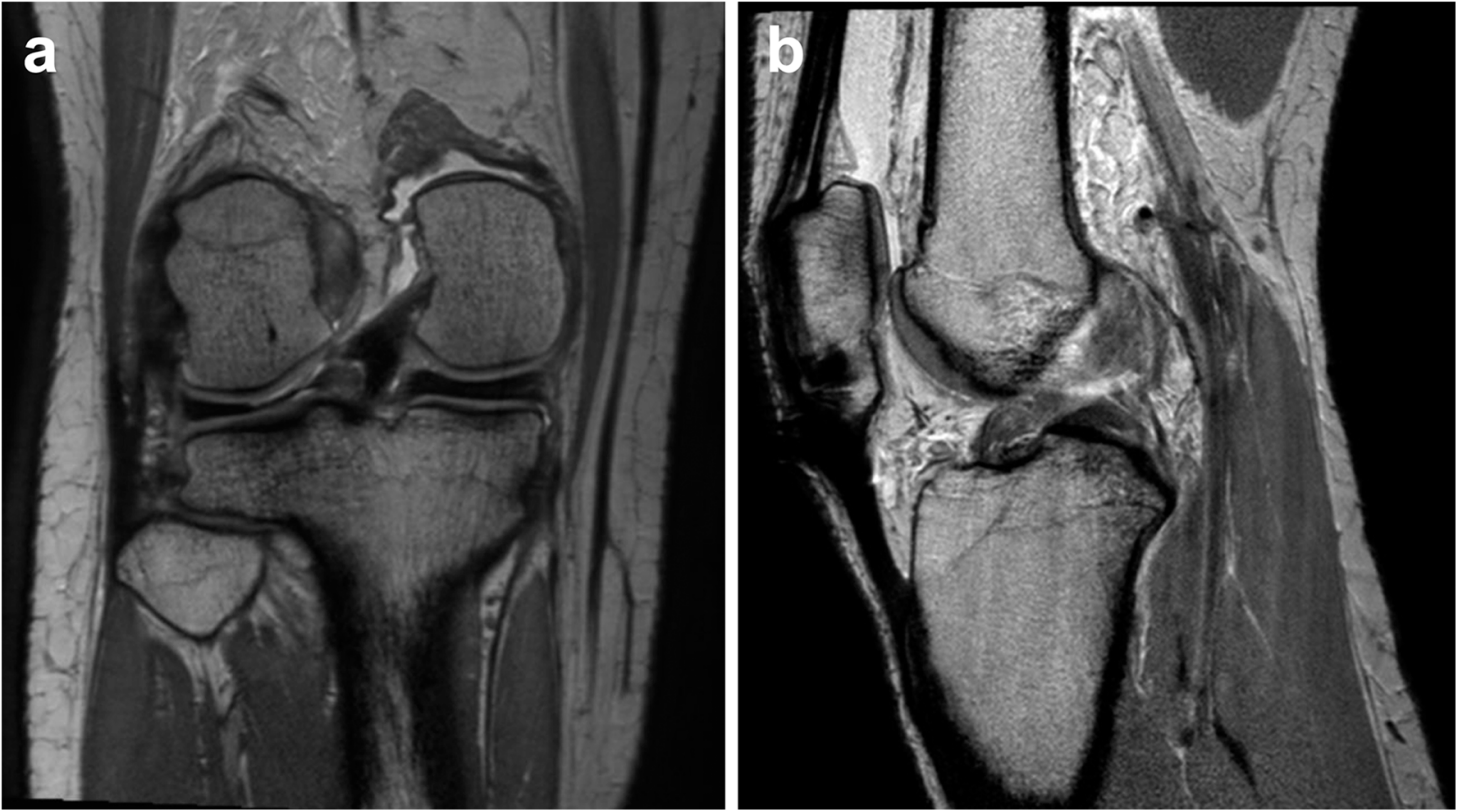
Knee MRI of a patient who had a lateral meniscus root tear. Proton-density-weighted coronal scan (a) showed increased signal intensity on the lateral meniscal root, suggesting a meniscal tear. Although there was a meniscal root tear, meniscal extrusion was not evident. In addition, there was an anterior cruciate ligament tear. Focal discontinuity in the mid-segment of the anterior cruciate ligament with diffuse swelling was seen in the proton-density-weighted sagittal scan (b).
